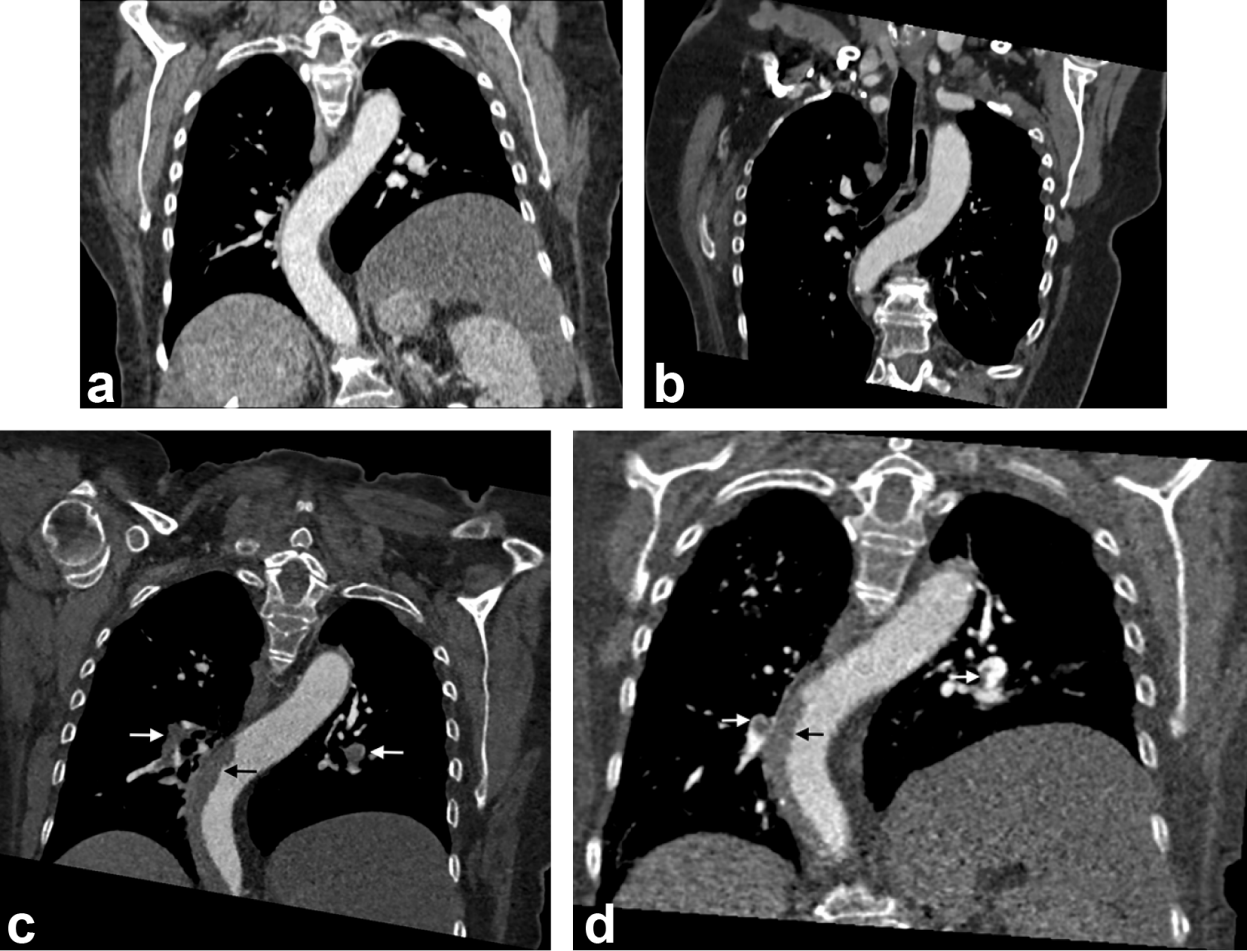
(a) Coronal CT image 2 months prior to admission showing no evidence of thrombosis in the thoracic aorta. (b) Staging CT 1 month prior to admission showing no evidence of thrombosis in the thoracic aorta. (c) Coronal CT image at the time of admission showing acute mural nonocclusive thoracic aortic thrombus (white arrow, pulmonary emboli; black arrow, aortic thrombosis). (d) Coronal CT image 3 weeks after commencement of rivaroxaban showing moderate reduction in aortic thrombosis and significant reduction of embolic burden (white arrow, pulmonary emboli; black arrow, aortic thrombosis).
